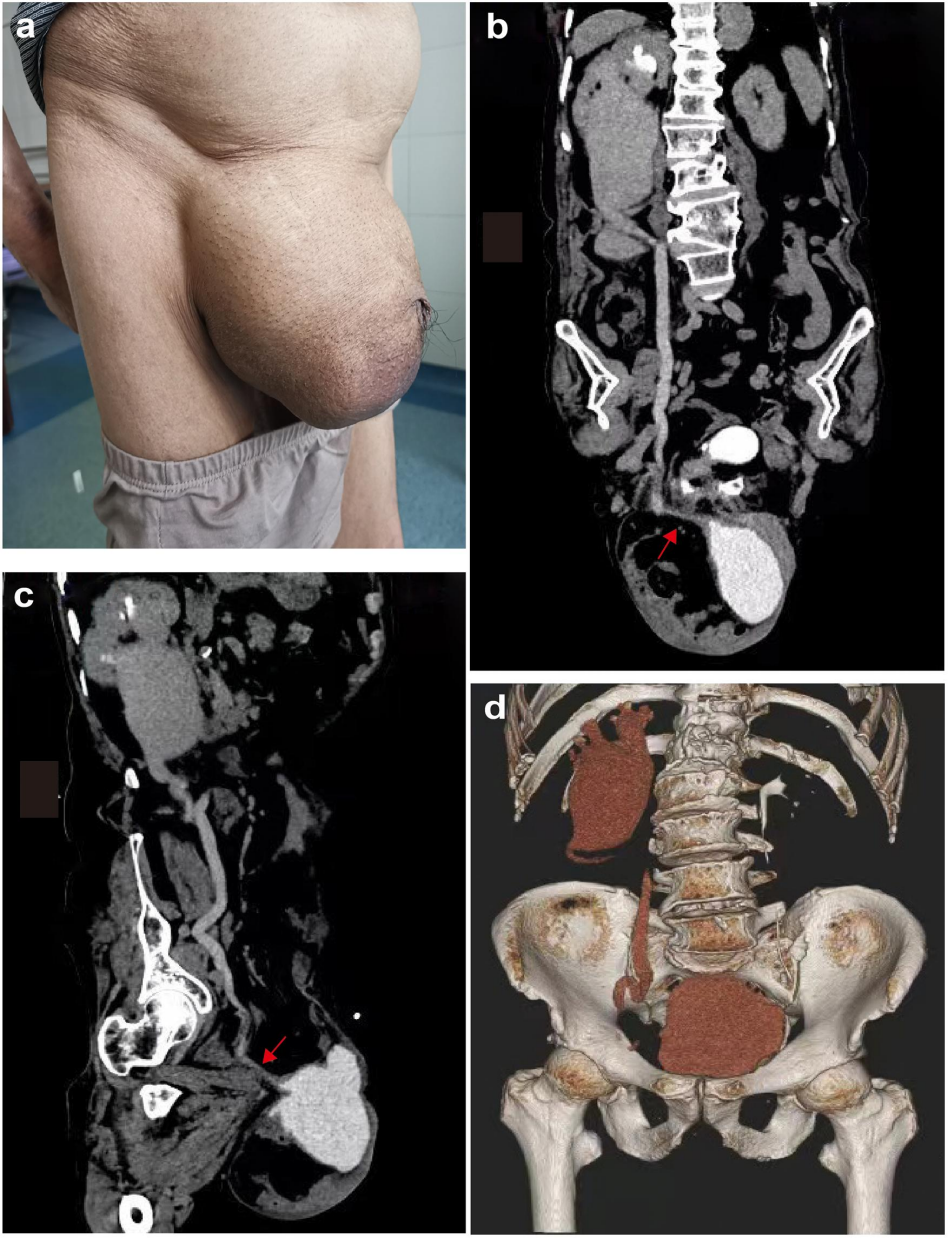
(a) Preoperative appearance of the right inguinal region (b,c) preoperative and postoperative CT urography (CTU) of the patient. CTU demonstrates a right inguinal hernia, intestinal and omentum hernia, bladder, and distal ureteral hernia, dilated right ureter, and severe right hydronephrosis. The red arrow marks the herniated right distal ureter. (d) The CTU was repeated 7 days after surgery, and the bladder and right ureter were repositioned.
